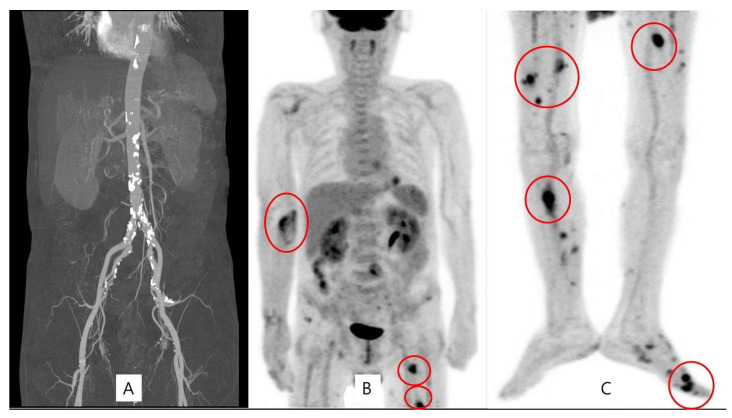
Normal aorta in abdominal CT (A) and multiple peripheral mycotic aneurysms were observed on the PET scan (B,C).
